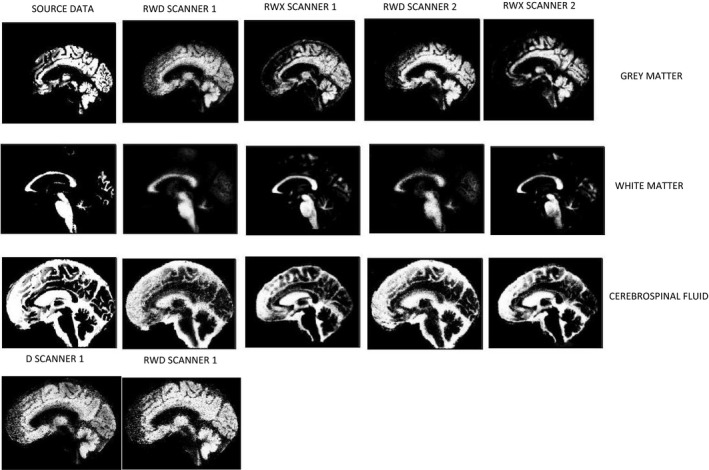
Comparison of tissue maps in patient space for the source magnetic resonance imaging (left) and after segmentation of acquisition C using the rewindowed default (RWD) method for scanner 1, RWD for scanner 2 then the rewindowed age‐specific maps (RWX) methods for each scanner. Top row is gray matter, second row is white matter, and third row is cerebrospinal fluid. It can be seen that the RWX segmentations are noticeably less blurred. The bottom row compares default segmentations with (RWD) and without rewindowing (D) for scanner 1, showing greater uniformity particularly around the motor/sensory cortex for RWD.
